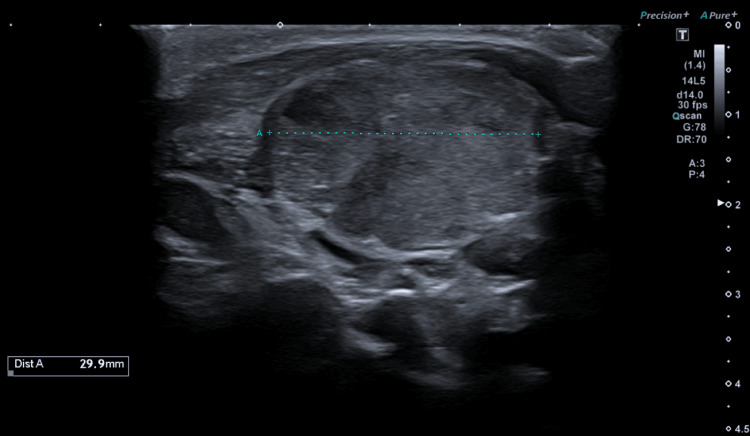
Ultrasound image of the right cervical swelling showing a large cervical mass (45x29.9x41.7 mm).
